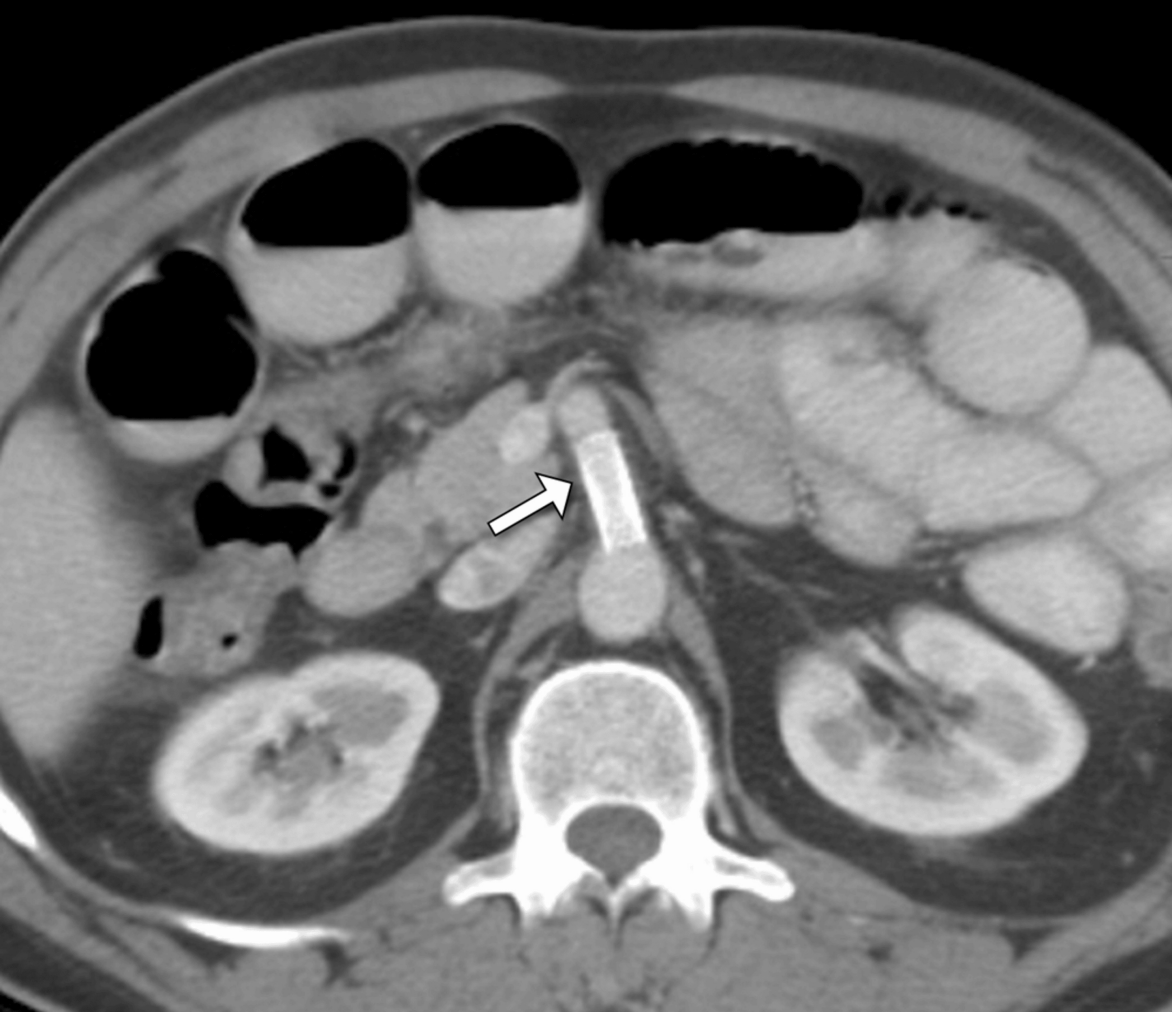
Axial CT demonstrating SMA stent placement Axial contrast-enhanced CT shows a metallic stent deployed within the SMA (arrow), with restored luminal caliber. There is no evidence of stent migration or perivascular complication. CT: computed tomography; SMA: superior mesenteric artery.
